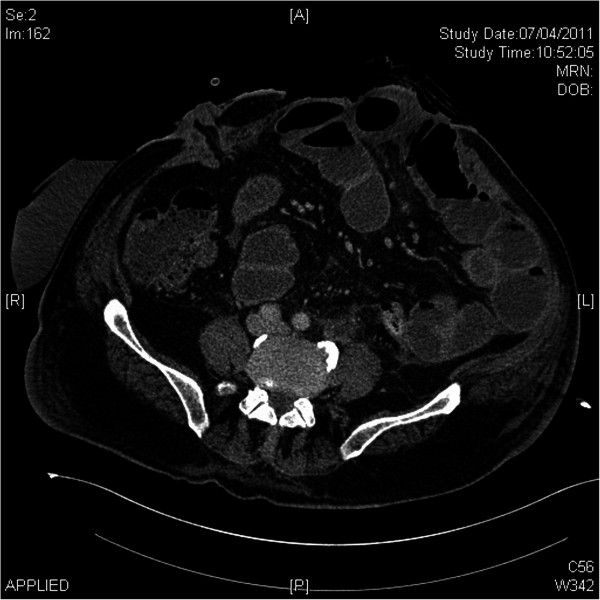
CT scan demonstrating a patient with a stoma and a large incisional hernia with loss of domain.
